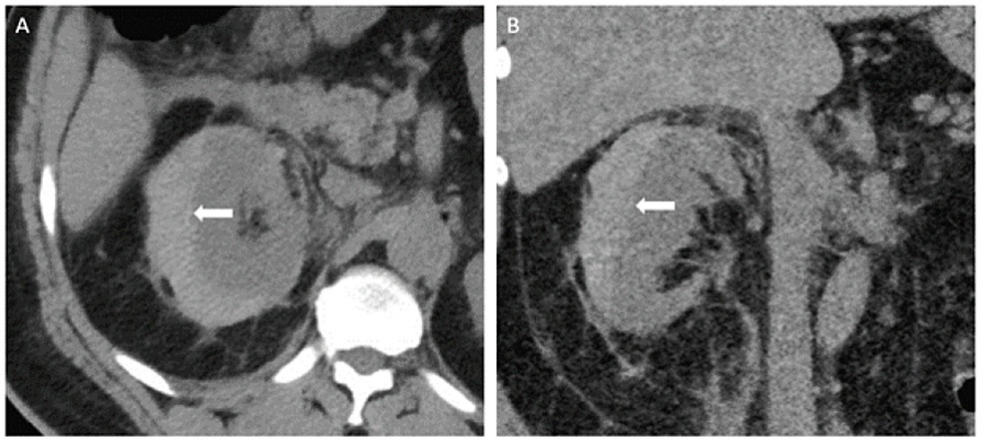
Initial imaging Axial (A) and coronal (B) non-contrasted CT scan of the abdomen/pelvis showing a subcapsular hematoma of the right kidney measuring up to 3 cm in thickness
